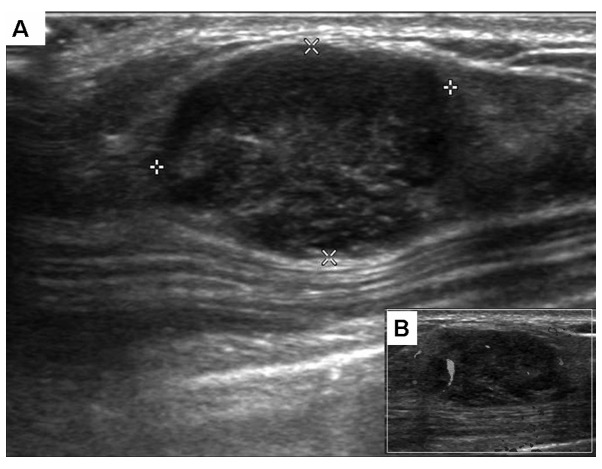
(A) Ultrasound shows a solid mass with heterogeneous internal echogenicity and a gently lobulated border. (B) Color Doppler ultrasound reveals an intralesional vascular flow.
